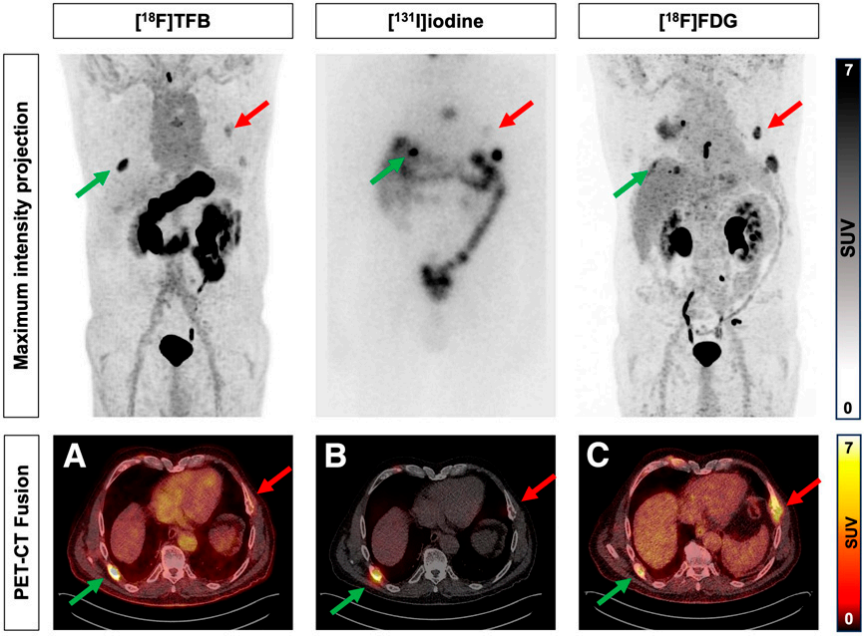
Heterogeneous disease pattern. 71-y-old man with follicular thyroid carcinoma and various bone metastases. Rib metastases show heterogeneous disease pattern: intense [^18^F]TFB (A) and [^131^I]iodine (B) uptake but low [^18^F]FDG (C) uptake (green arrow); intense [^18^F]FDG (C) uptake but no relevant [^18^F]TFB (A) and [^131^I]iodine (B) uptake (red arrows).
